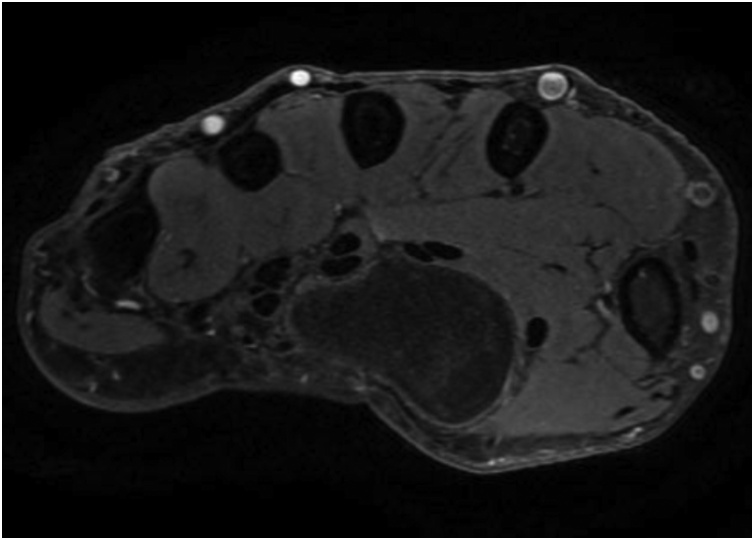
Axial MRI image with fat suppression showing an occupying-space lesion compatible to adipose tissue.
